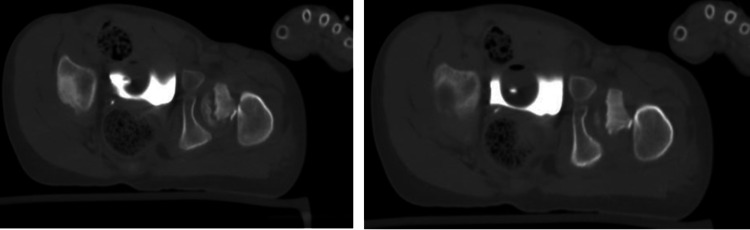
CT scan of the pelvis shows neck of femur fracture
